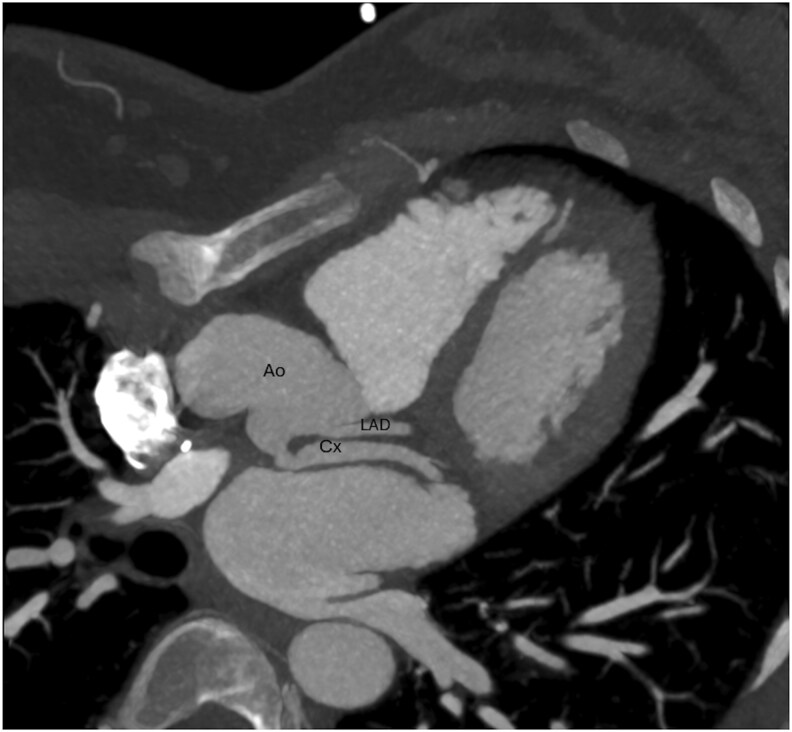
Post-operative CT showing the patent circumflex coronary artery (Cx) after re-implantation into the aorta (Ao) behind the left anterior descending artery (LAD).
